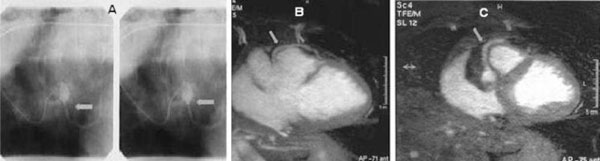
(A) Conventional x-ray angiography showing an anomalous originating left main coronary artery (LMCA, arrow) from the right aortic sinus. (B, C) Transverse MR angiographic images showing an anterior course of the LMCA in relation to the pulmonary trunk (arrow).
